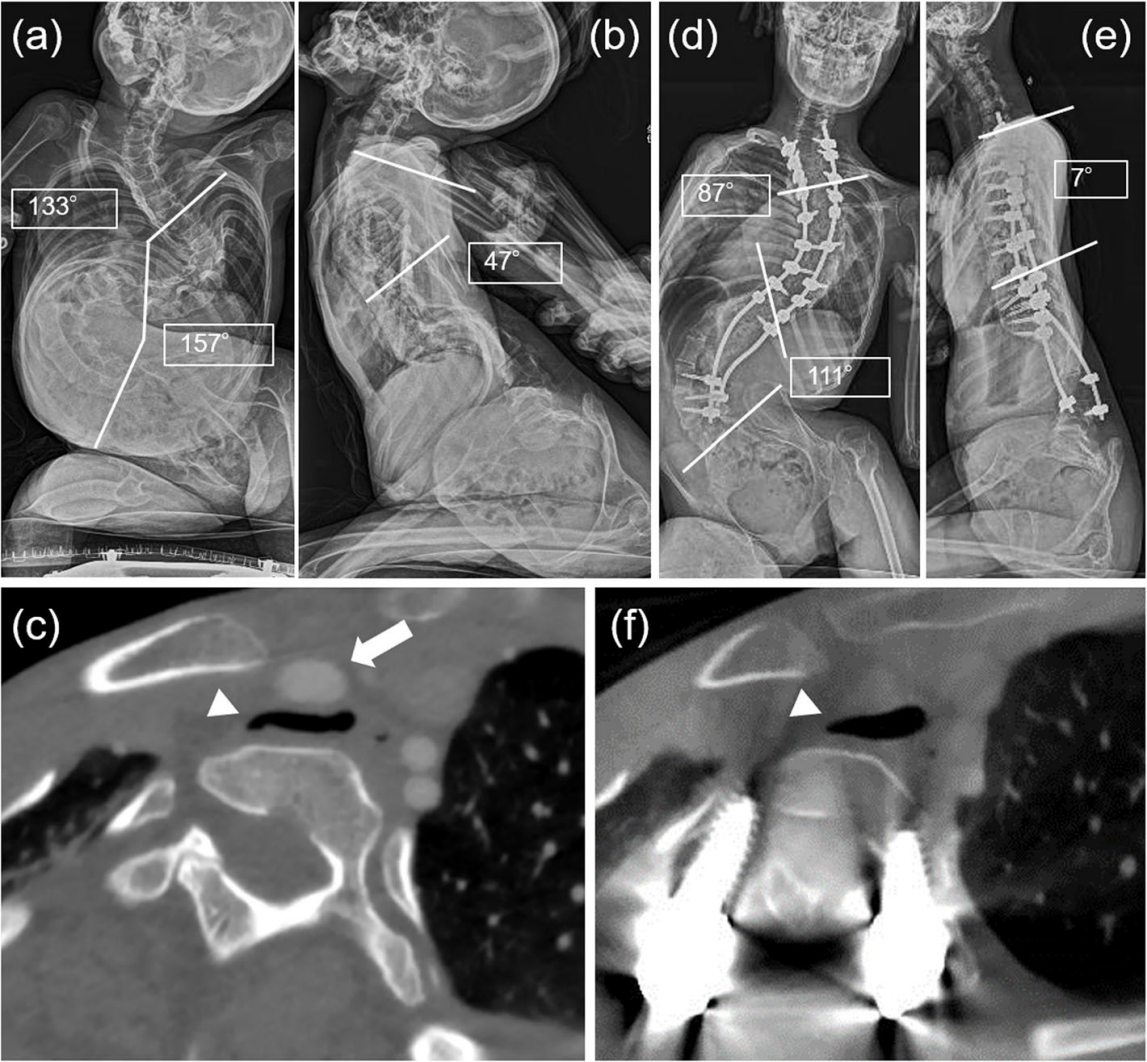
Image findings of case 1. a, b Preoperative posterior-anterior and lateral X-rays of the whole spine in assisted sitting position shows severe scoliosis with exaggerated cervicothoracic hyperlordosis. c Preoperative contrast enhanced computed tomography at T2 demonstrates a flattened tracheal lumen between innominate artery and anteriorly deviated vertebra. d, e Postoperative posterior-anterior and lateral X-rays of the whole spine in assisted sitting position reveals well-corrected cervicothoracic alignment. f Postoperative computed tomography demonstrates the expansion of the narrowed tracheal lumen.The white arrow in (c) indicates the innominate artery compressing the trachea from the anterior aspect. The white arrowheads in (c) and (f) indicate the tracheal lumen
